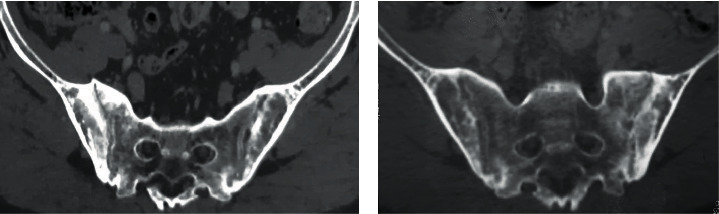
CT scan 12 months later showing ankylosis of the sacroiliac joint.
